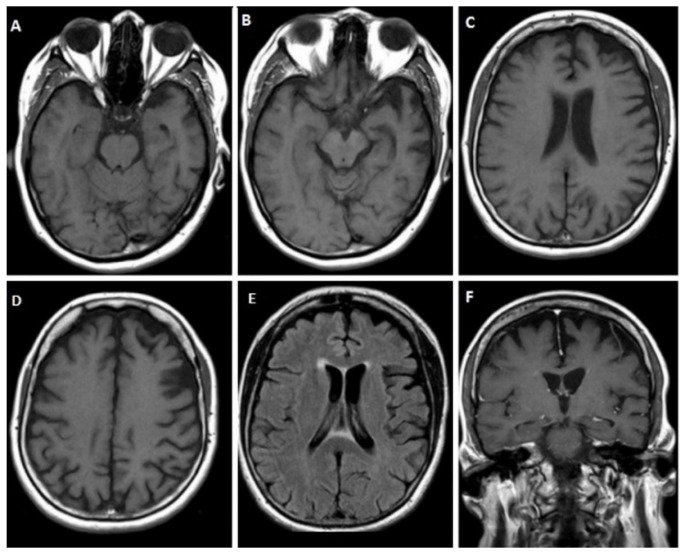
Brain MRI of case 1. T1 sequence (A–D) and fluid attenuated inversion recovery (FLAIR) (E) showing cortical atrophy in the frontal and temporal lobes, with left hemisphere being more affected, as well as coronal T1 section (F) showing relative preservation of the hippocampus.
